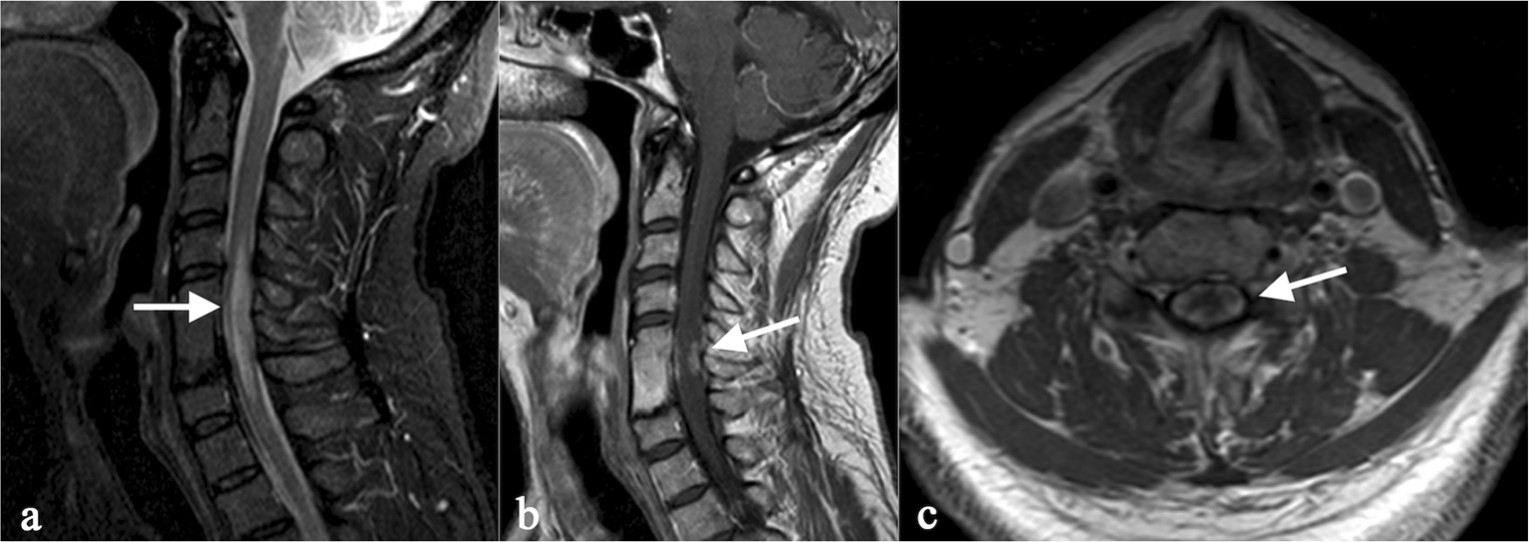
MRI images show spinal cord involvement in a 54-year-old man. T2-weighted image (Fig. 15a) demonstrates an extensive area of high signal intensity in the spinal cord (*white arrow*). On enhanced T1-weighted acquisitions, a peripheral enhancement (*white arrows*) can be depicted (Fig. 15b and c), suggesting meningeal involvement
